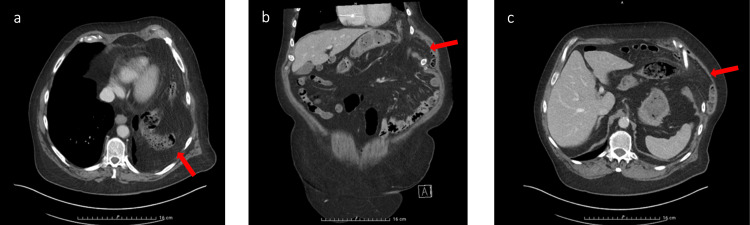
Left transdiaphragmatic intercostal hernia: preoperative thoracoabdominal CT scan imaging. a. Thoracic sagittal view b. Frontal thoracoabdominal view c. Abdominal sagittal view
